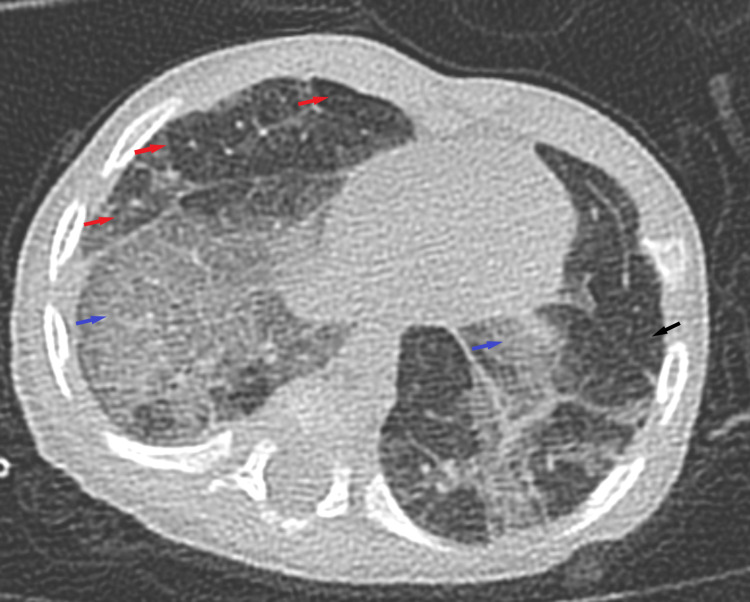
Chest CT scan with contrast. Chest CT with contrast showed mosaic attenuation pattern in both lower lungs (red arrows), bilateral ground-glass opacities with areas of reduced attenuation (blue arrows), areas of oligemia, and air-trapping (black arrow).
